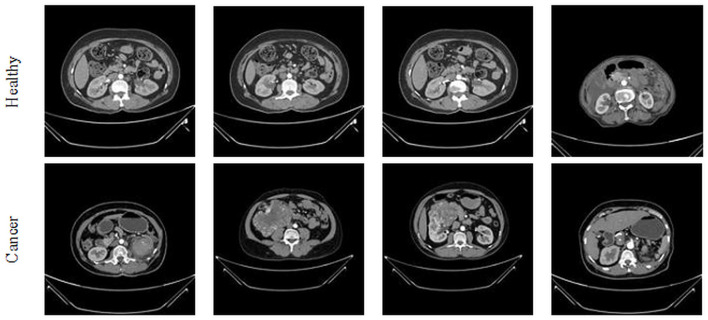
Sample axial-plane test images of renal CT slices.
